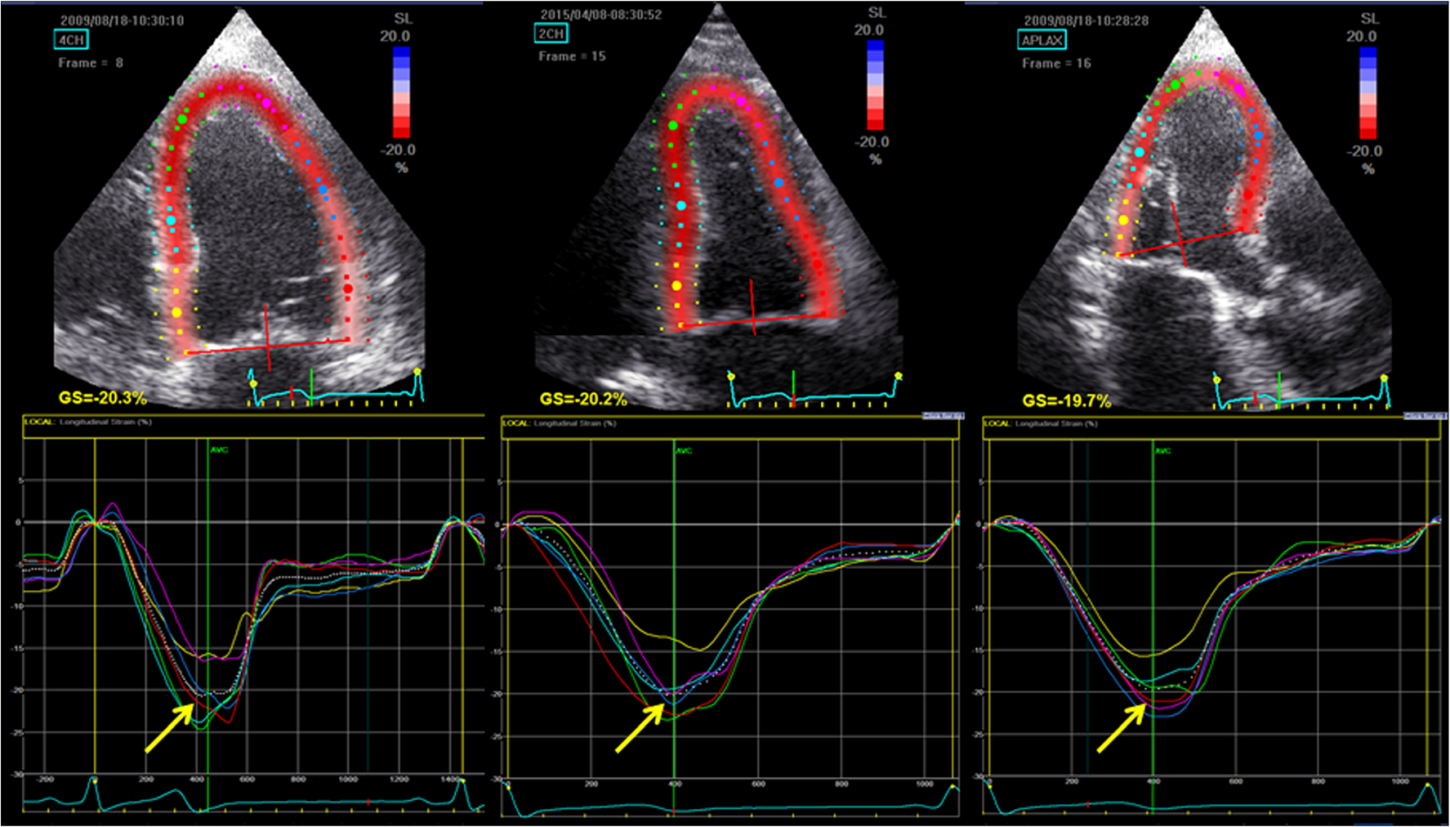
Example of calculation of global longitudinal strain (GLS) by 2D speckle tracking for the apical four-, two-, and three-chamber views. The strain throughout one cardiac cycle can be seen for each of the colour-coded LV segments (mean strain shown in *white*). In this example, the mean peak strains are *−20.3*, *−20.2*, and *−19.7 %* (indicated by the *arrows*), which occur during LV ejection. The calculated GLS is −20.1 %; a more negative strain indicates better systolic function. AVC aortic valve closure
